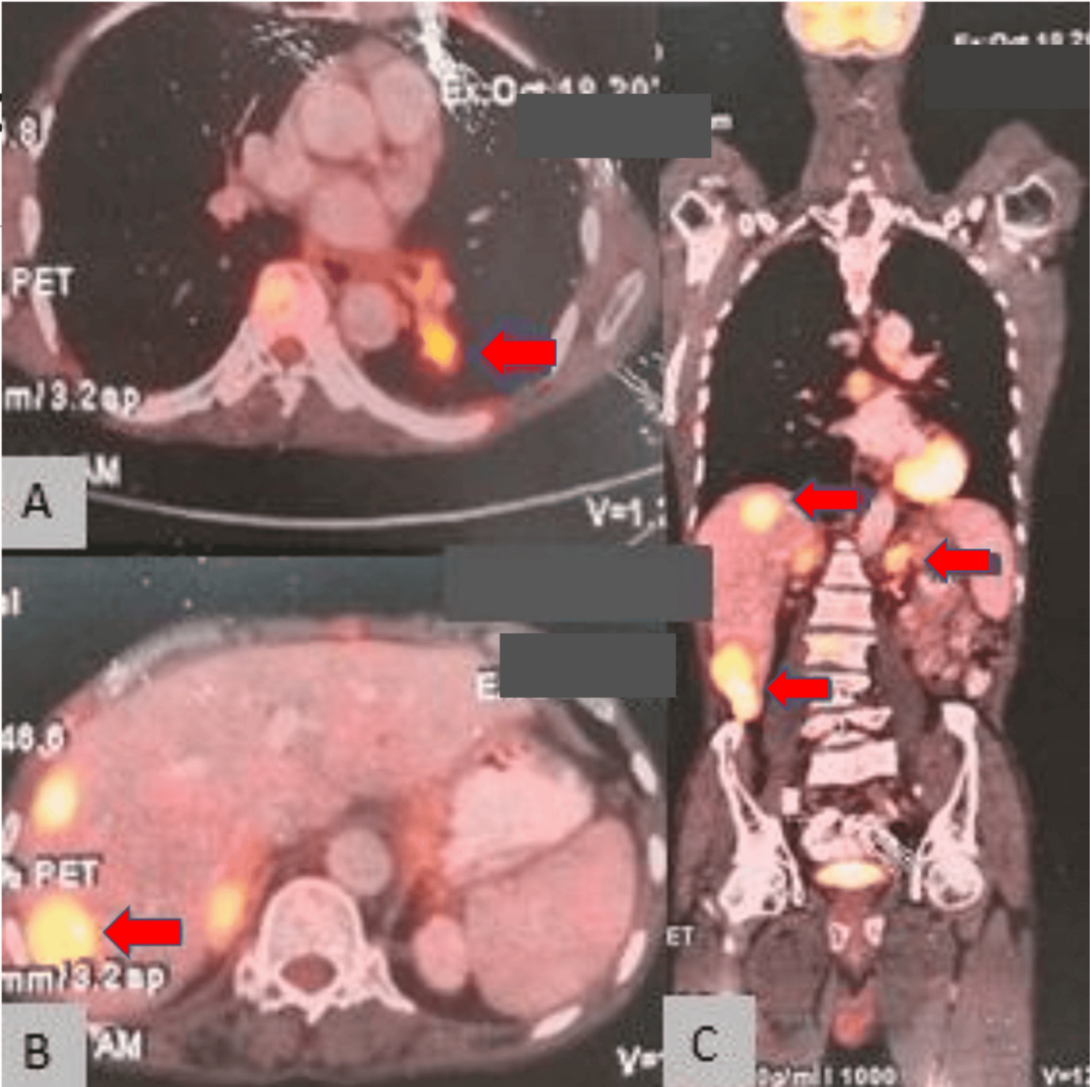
On Fluoro-deoxyglucose-positron emission tomography (FDG-PET), a cross-sectional view depicting intense uptake in a nodular mass lesion in the left lower lung lobe (A). Cross-sectional view depicting intense uptake in the liver nodules (B), a longitudinal section depicting intense uptake in the brain lesions, and a few retroperitoneal lymph nodes (C).
